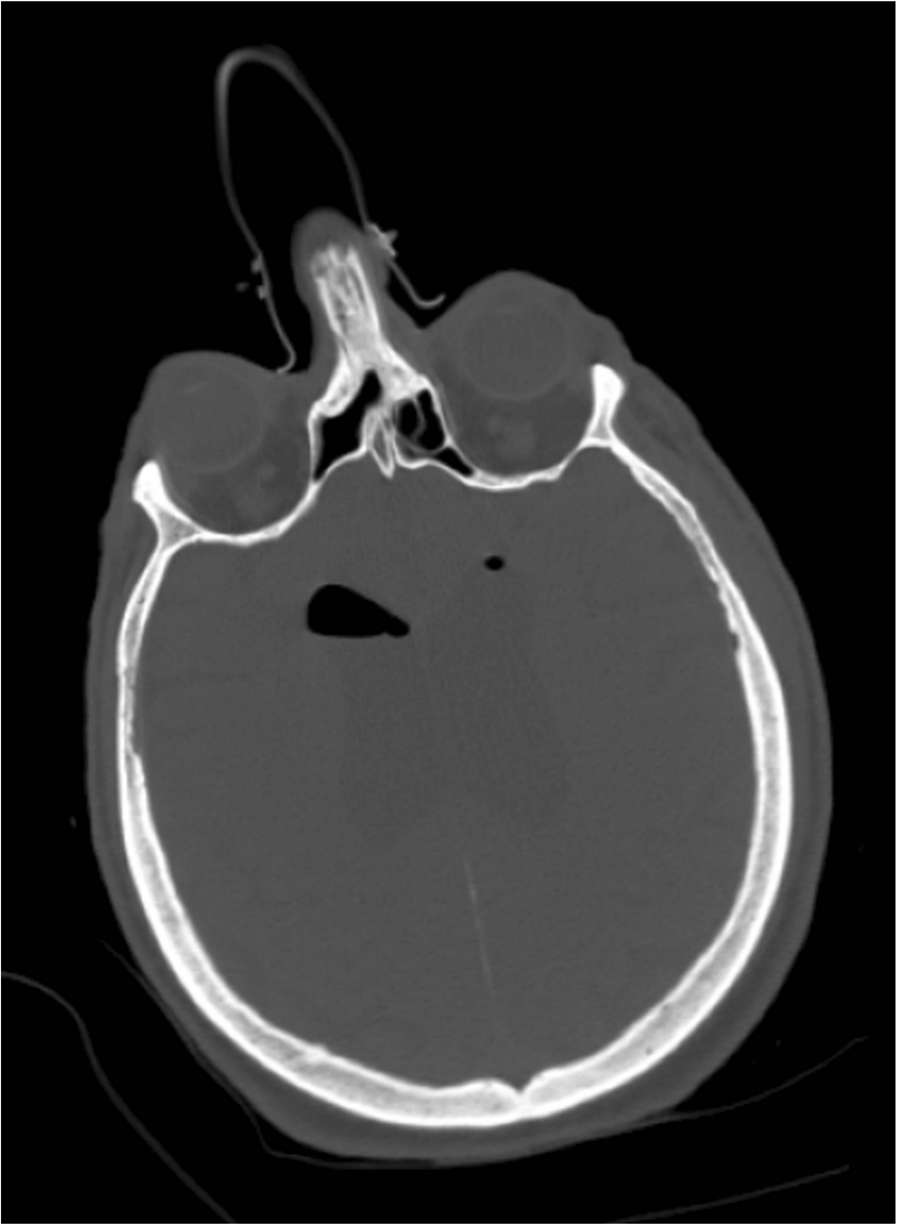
Initial brain computed tomography scan, axial view, showing pneumocephalus inside the lateral ventriculi. There are no associated fractures of the skull or the sinus walls
